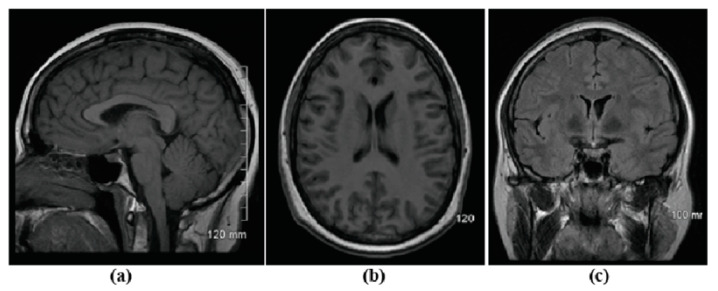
Brain MRI obtained from (a) Sagittal Plane, (b) Axial plane, and (c) Coronal plane.
